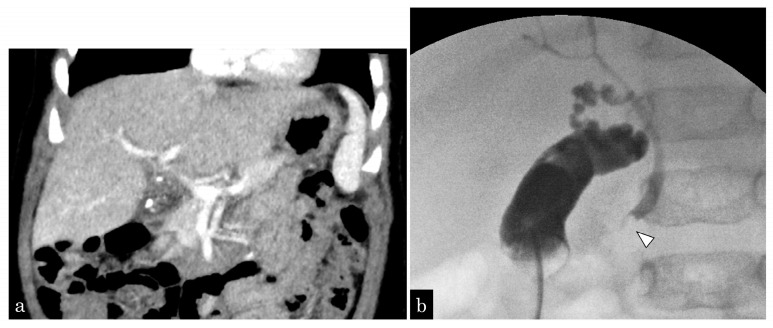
A 5-month-old girl presented with vomiting and revealed gallbladder and common bile duct stones on ultrasonography. (a) Enhanced computed tomography (CT) showing multiple small gallstones and gallbladder swelling. (b) The patient developed septic shock due to cholangitis. Emergency gallbladder drainage was performed, and a bile stone impacting the distal common bile duct (white arrowhead) was noted. A 4-Fr ENBD tube was inserted.
